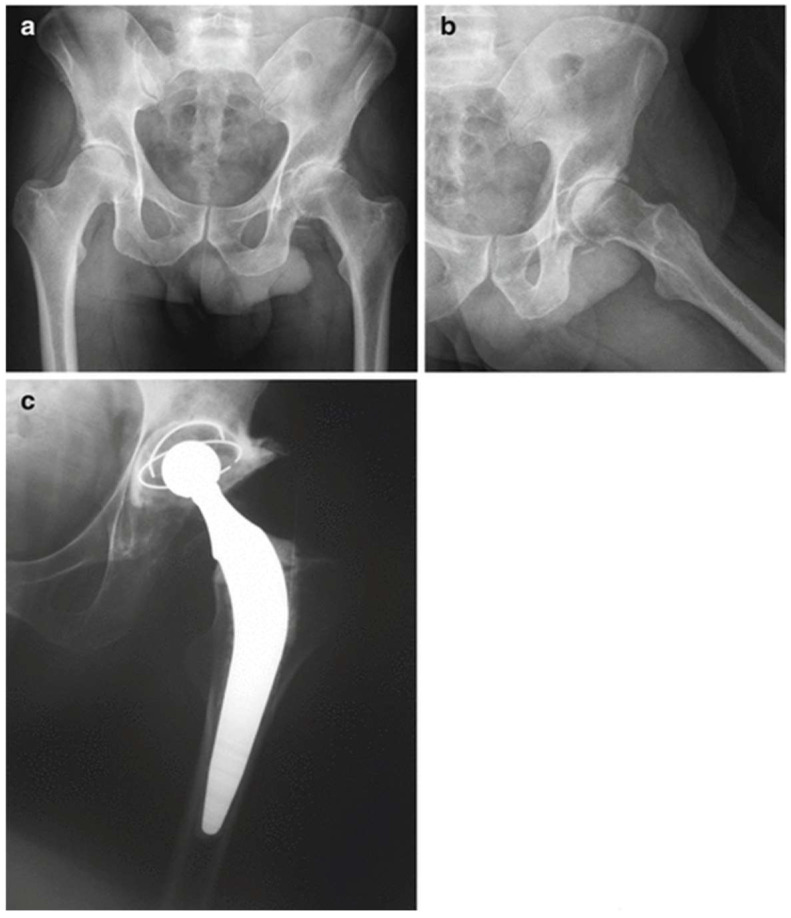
Total hip replacement (THR) in a haemophilia patient performed without using pharmacological thromboprophylaxis. Anteroposterior preoperative radiograph (a). Lateral preoperative view (b). Postoperative radiograph (c).
